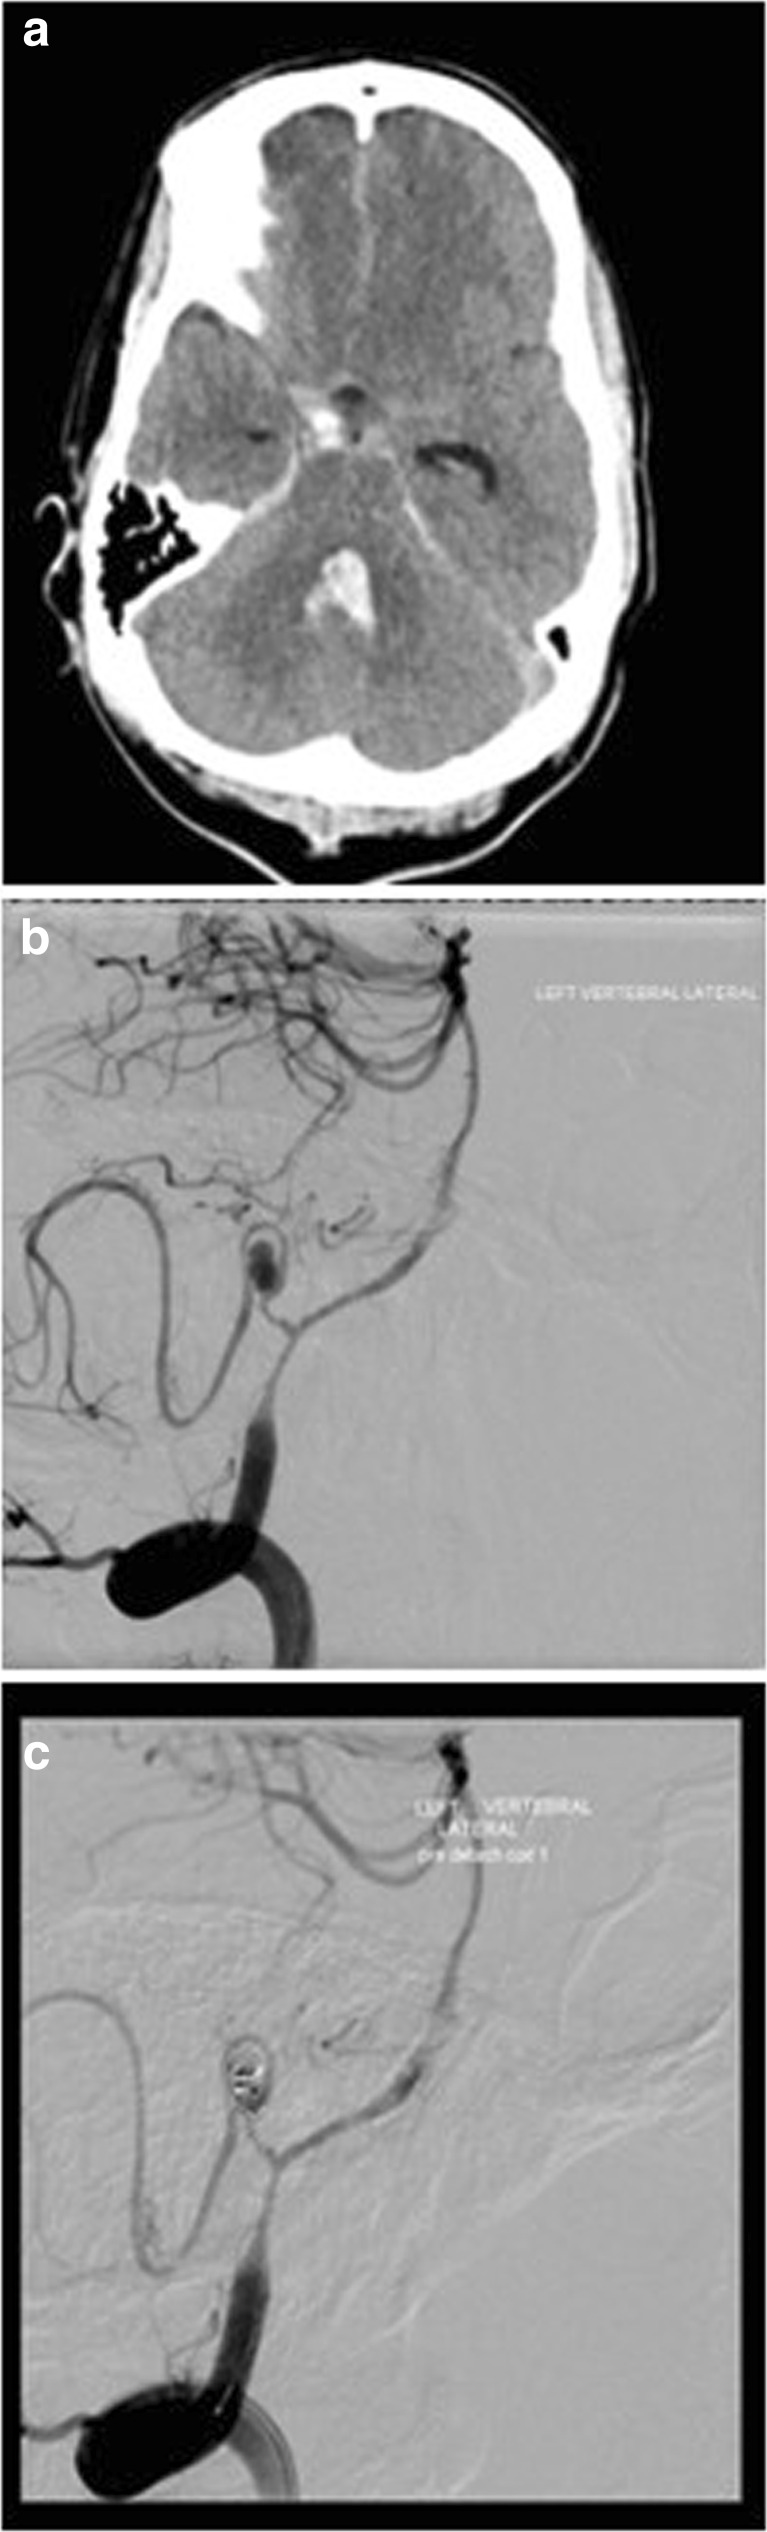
a CT demonstrating diffuse subarachnoid blood and intraventricular haemorrhage with hydrocephalus. b DSA demonstrating left PICA. c Post coiling DSA demonstrating coil occlusion of the left PICA TICA
